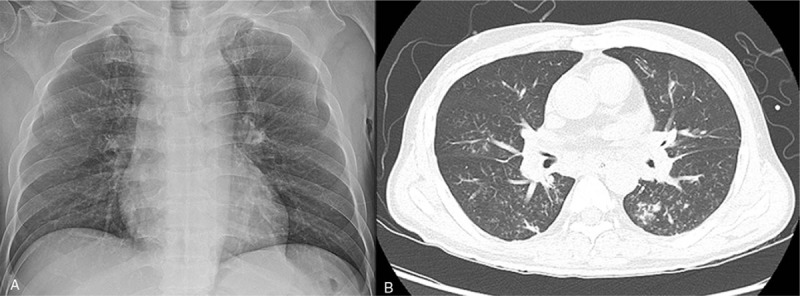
Initial chest radiograph and CT. (A) Chest radiograph at emergency room arrival. (B) Chest CT showing centrilobular nodules with linear branching opacities and confluent consolidation in both the lungs. CT = computed tomography.
